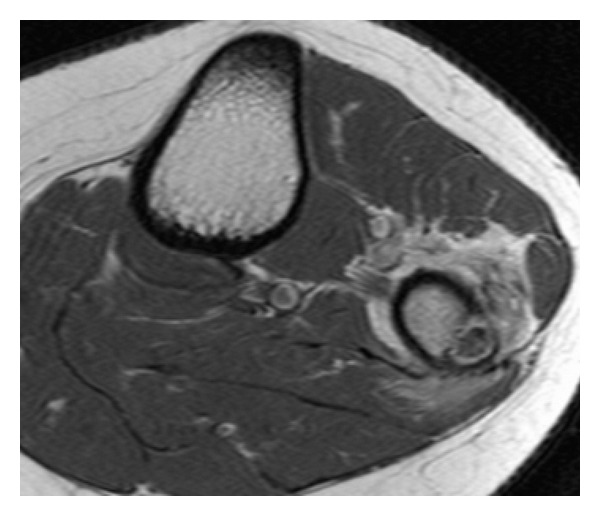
T1-weighted MRI scan showing the nidus surrounded by hyperintense tissue.
